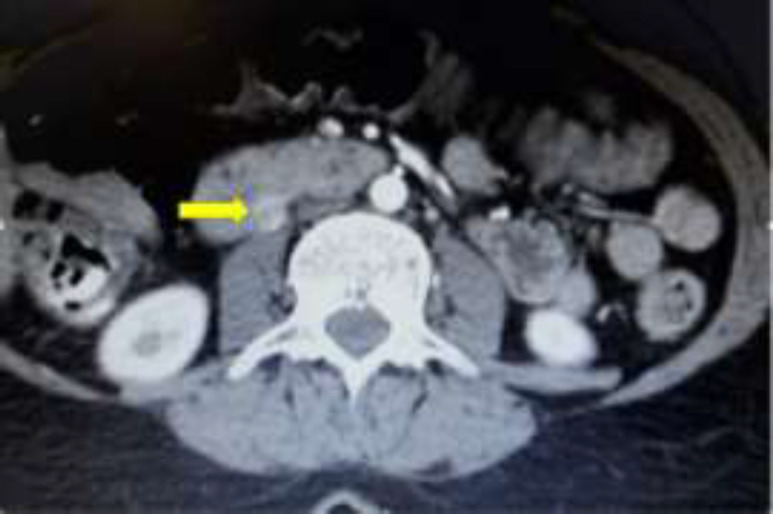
Nodular lesion in the posterior wall of third duodenal in abdominopelvic CT scan
